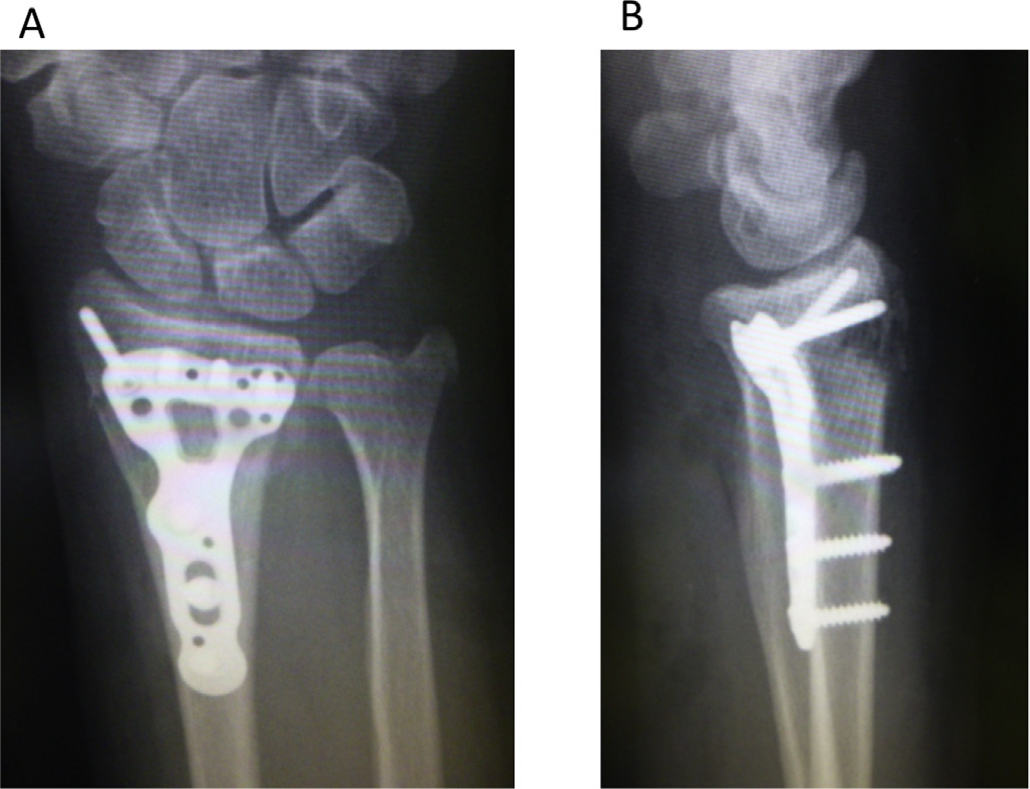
Plain radiography after the first osteosynthesis. Osteosynthesis was applied to the distal radius fracture using a volar locking plate (A: frontal view, B: lateral view).
